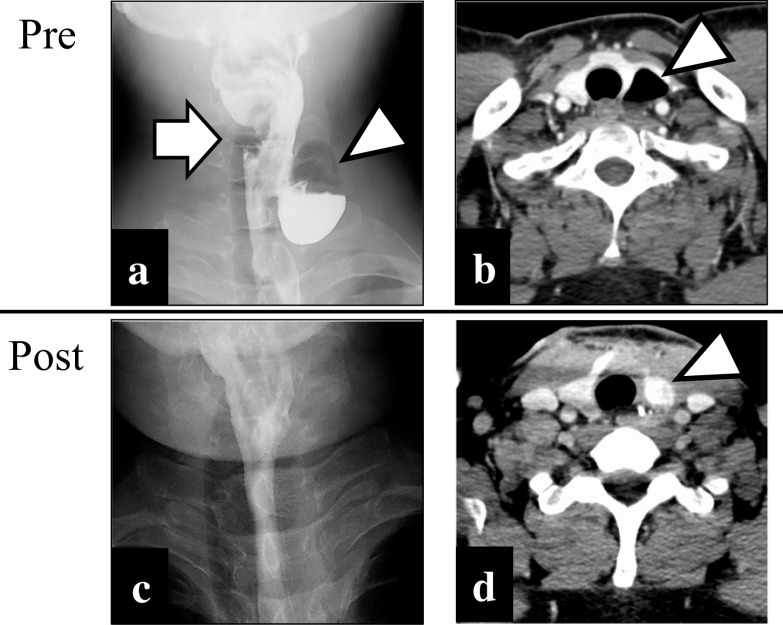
Preoperative and postoperative findings. a Preoperative esophagography showing a left-sided outpouching sac just below the cricopharyngeal bar. Arrow: cricopharyngeal bar; arrowhead: diverticulum. b Preoperative computed tomography with contrast showing a diverticulum (arrowhead) from the esophagus at the level of the cricothyroid cartilage. c Postoperative esophagography reveals no leakage. d Postoperative enhanced computed tomography showing that the thyroid gland flap (arrowhead) is accurately placed to cover the defect, and the surgical area is clearly enhanced.
